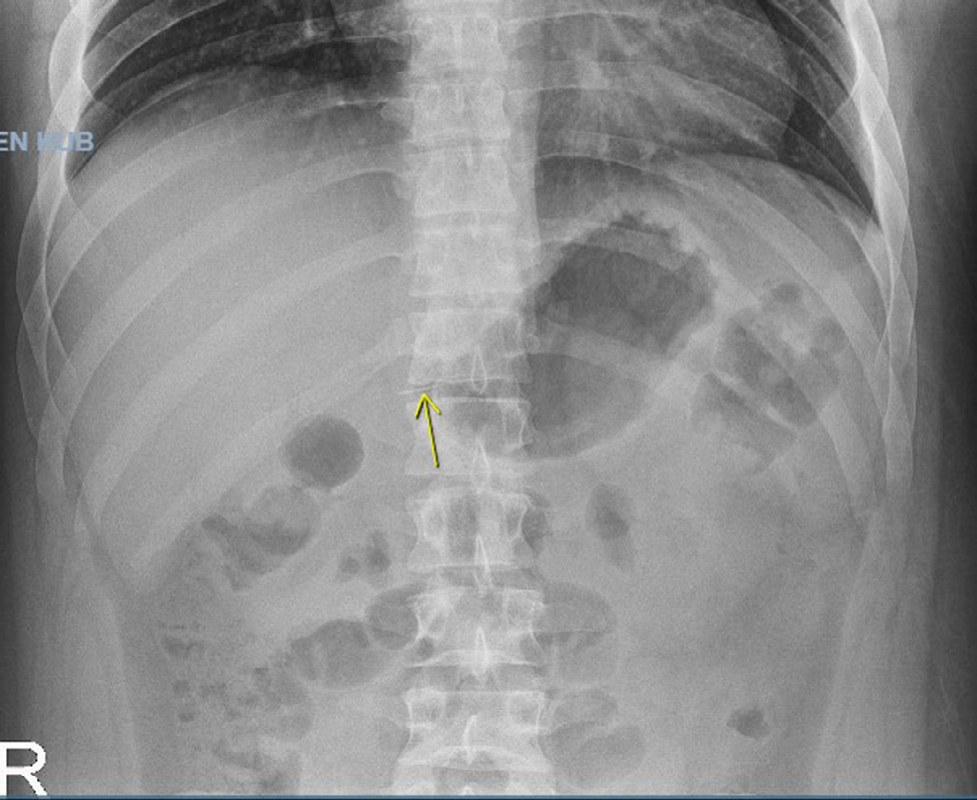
X-ray of abdomen demonstrating foreign object in the gastric antrum. Initial X-ray of the kidneys, ureters and bladder revealed a 4 mm linear radiopaque foreign body overlying the midline of the upper abdomen. Arrow indicates foreign body likely in the gastric antrum. No free air or obstruction identified.
